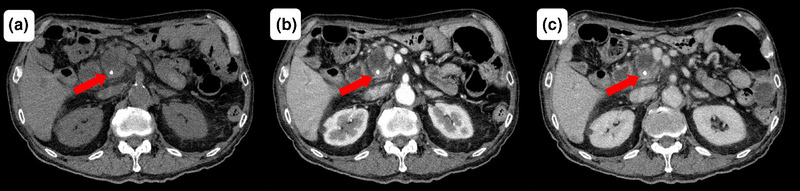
Contrast‐enhanced computed tomography findings before endoscopic retrograde cholangiopancreatography. Contrast extravasation is not observed before the replacement of the plastic stent (red arrow). (a) Plain computed tomography image. (b) Delayed arterial phase. (c) Portal phase.
